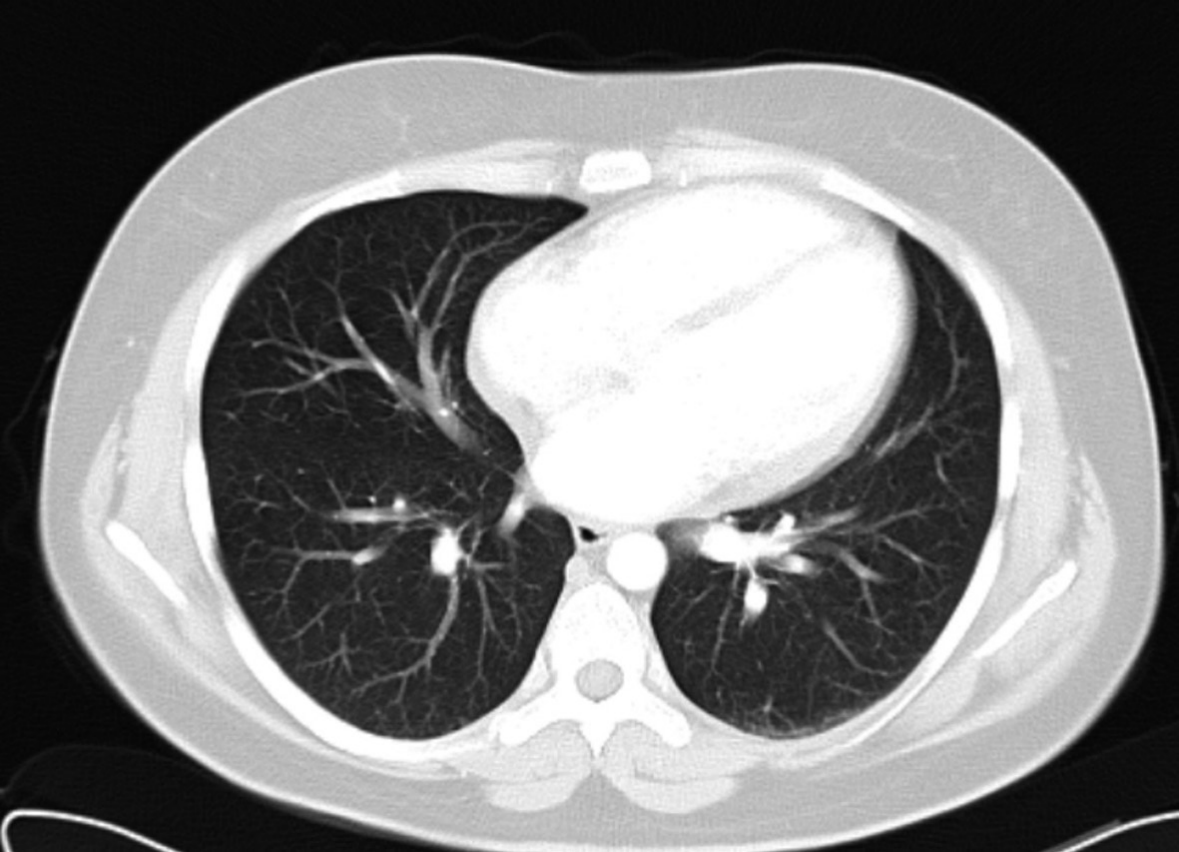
Contrast-enhanced chest computed tomography in the axial plane, showing no pericardial involvement.
